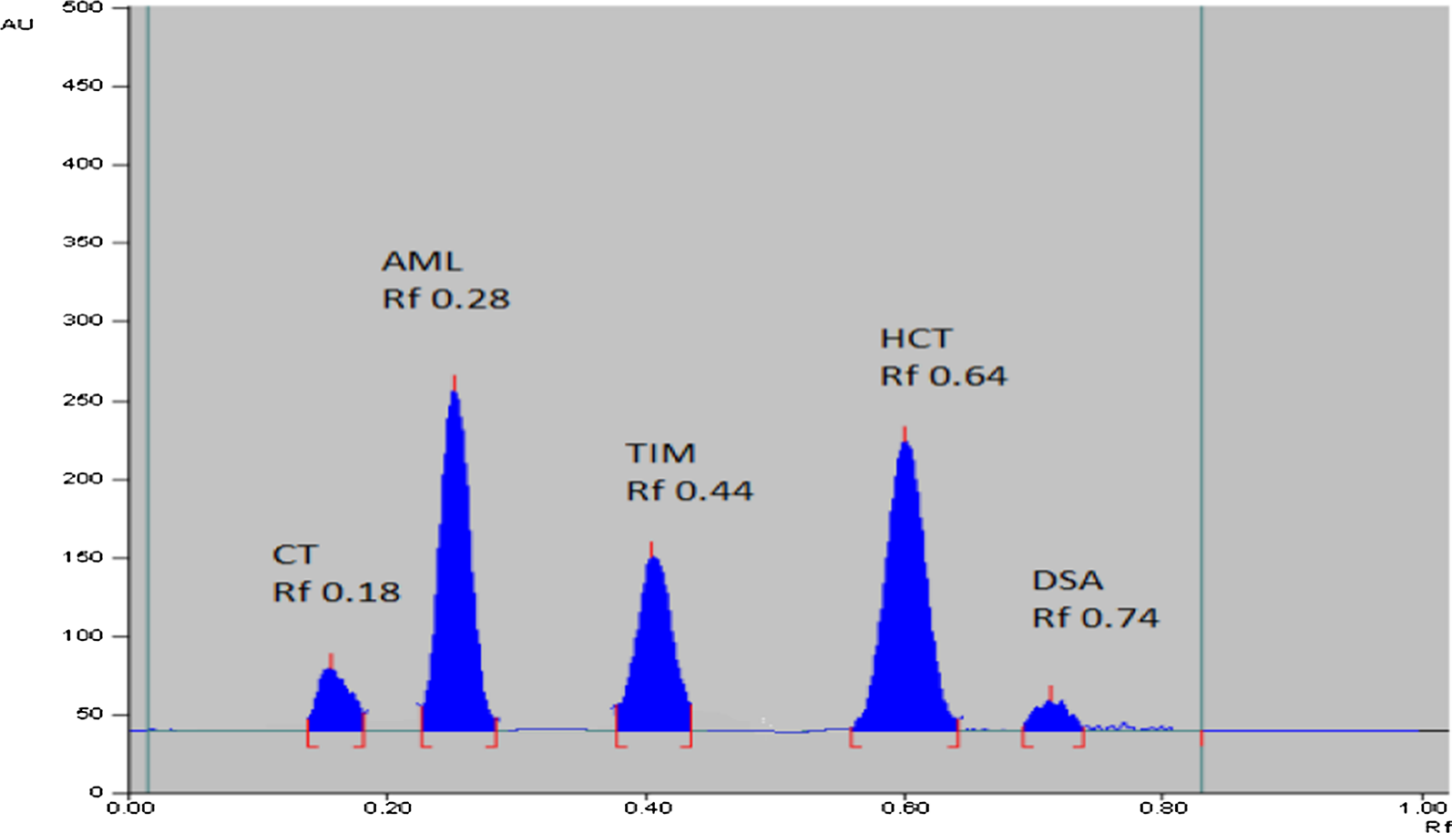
HPTLC-densitogram of mixture of AML (1.0 µg/band), TIM (1.0 µg/band), HCT (1.0 µg/band), DSA (0.05 µg/band), CT (0.05 µg/band) using ethyl acetate–ethanol–water–ammonia (8.5:1.0:0.5:0.3, by volume) as a developing system at 220.0 nm
